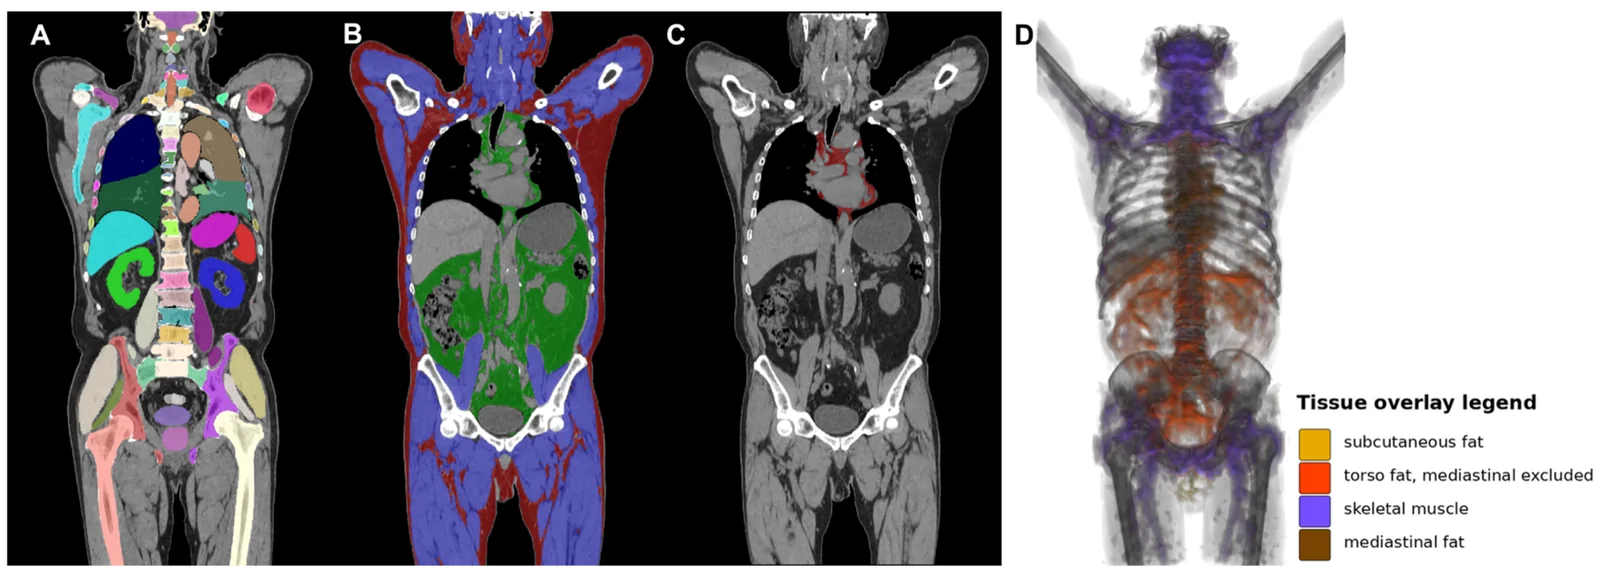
Representative PET/CT segmentation for organ and body composition analysis. (A) Multiorgan segmentation on coronal CT images. (B) CT-derived body composition segmentation, including SAT, SM, and TF. (C) Detailed visualization of MedF segmentation. (D) Three-dimensional rendering of the segmented body composition compartments. SAT, subcutaneous adipose tissue; SM, skeletal muscle; TF, torso fat; MedF, mediastinal fat.
